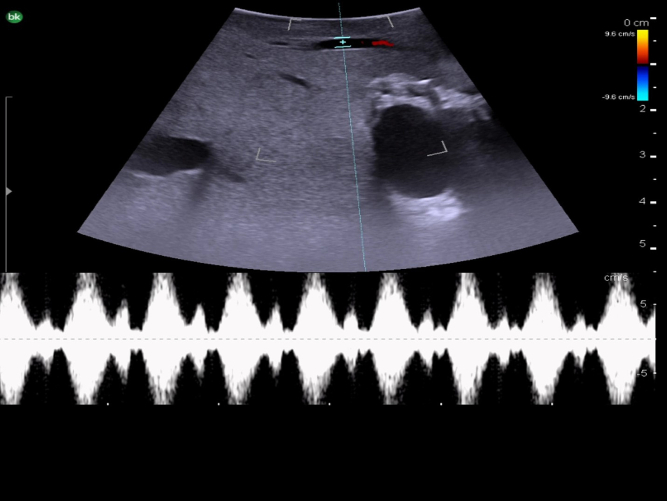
Intraoperative Doppler ultrasound of hepatic arterial flow.
